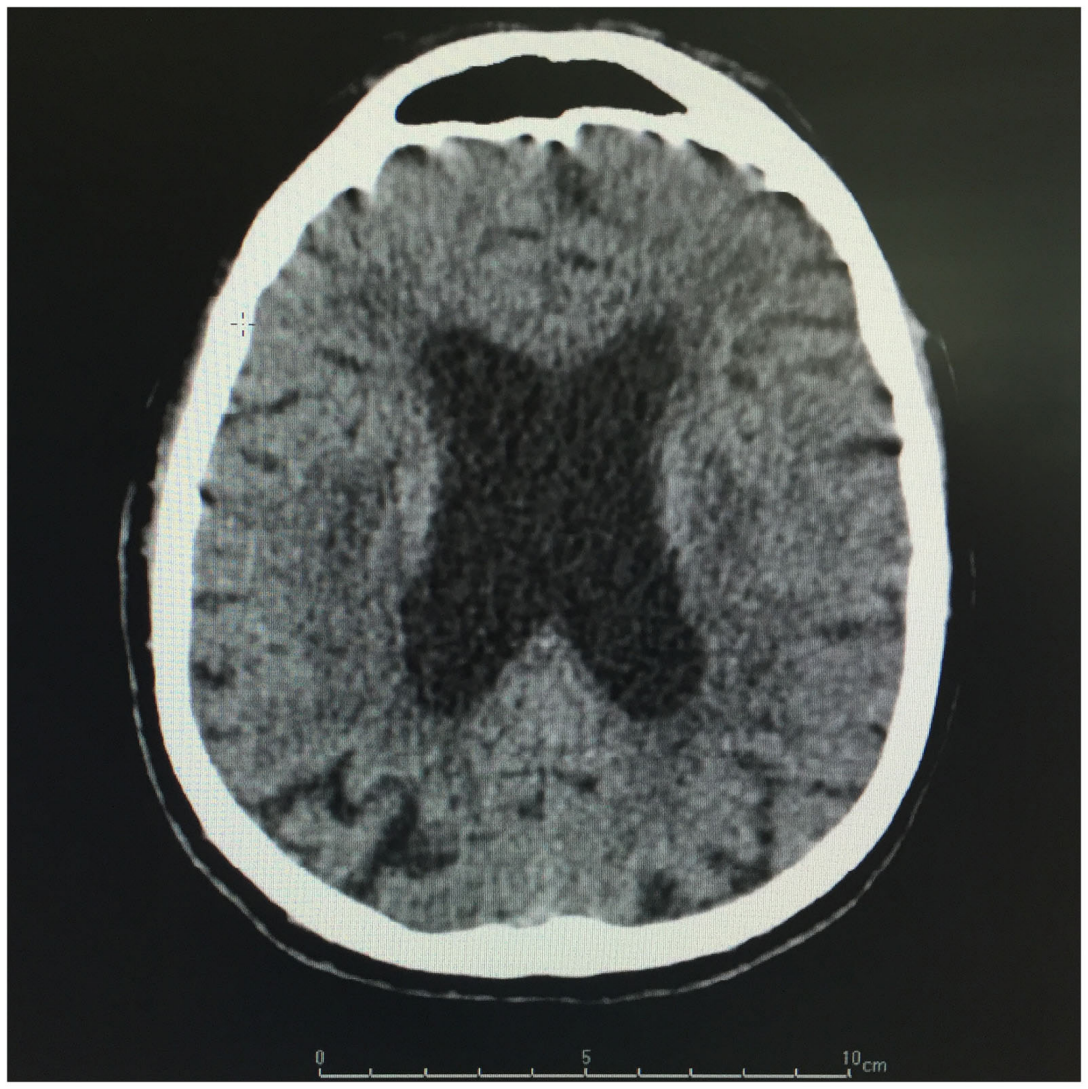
Brain CT: Lateral ventriculomegaly and periventricular hypointensities.
